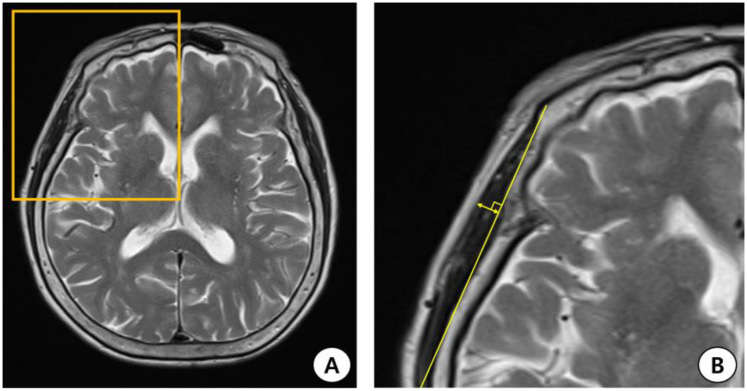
Process of measuring temporal muscle thickness (TMT) on the brain magnetic resonance image (MRI). (A) Axial T2-weighted MRI at the orbital roof level. (B) The Sylvian fissure is defined as a reference point in the anterior–posterior orientation. TMT was measured perpendicular to the long axis of the temporal muscle.
